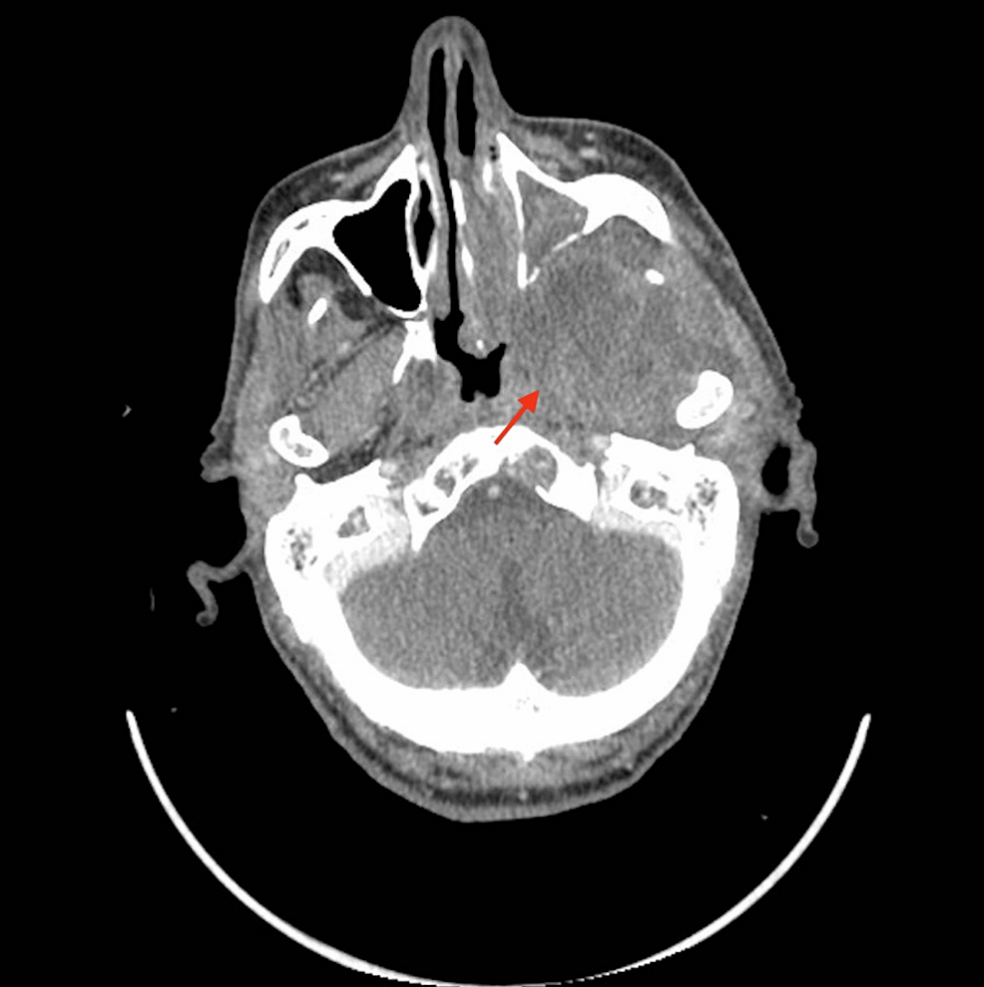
A CT scan performed six months after the end of radiation therapy showed a stable disease, with a residual lesion that extends to the infra-temporal fossa (arrow).
